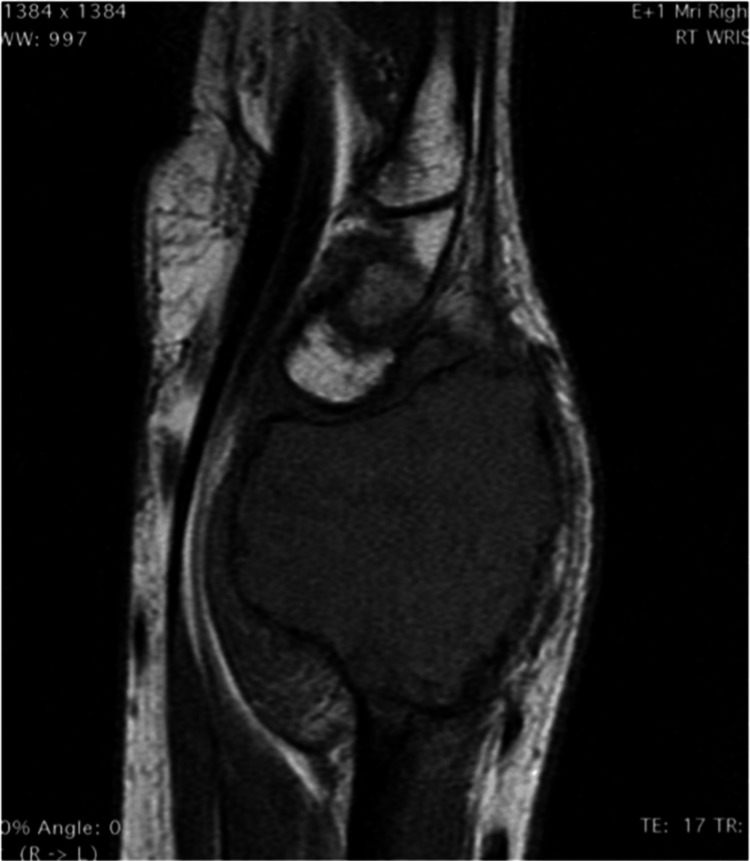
Gadolinium enhanced T1-weighted sagittal MRI image showing a low signal expansile lesion of the distal radius.
